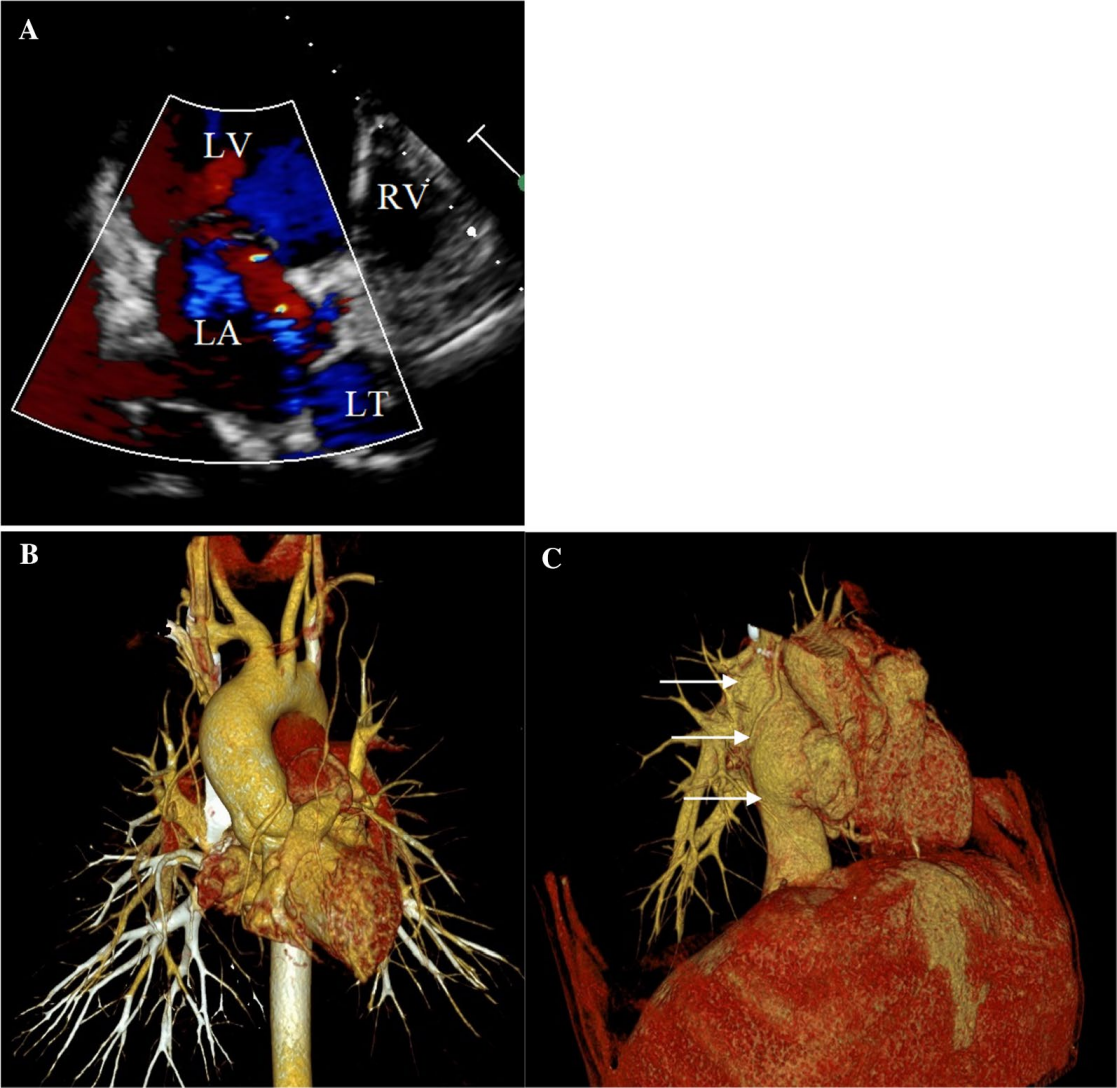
Echocardiogram and computed tomography. A 4 chamber view (LV—left ventricle, RV—right ventricle, LA—left atrium, LT—lateral tunnel), B anterior view, tricuspid atresia, C lateral view, total cavopulmonary anastomosis (lateral tunnel, white arrows)
